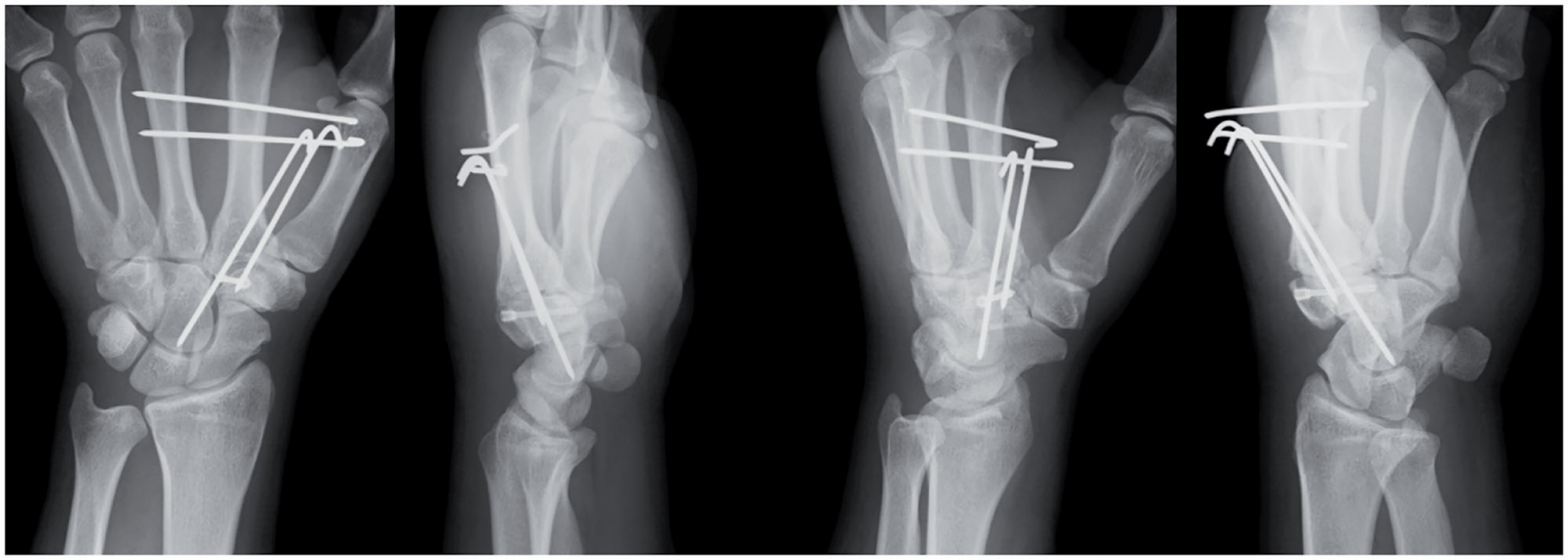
Postoperative X-ray images after surgical fixation of an isolated left trapezoid coronal shearing fracture in a 40-year-old male. The fracture was reduced and fixed with a double-threaded screw (DTJ mini screw, Meira Co., Ltd., Nagoya, Japan; width proximal 3.4 mm, distal 2.7 mm, length 20 mm). Kirschner wires were used to reduce the pressure on the trapezoid.
